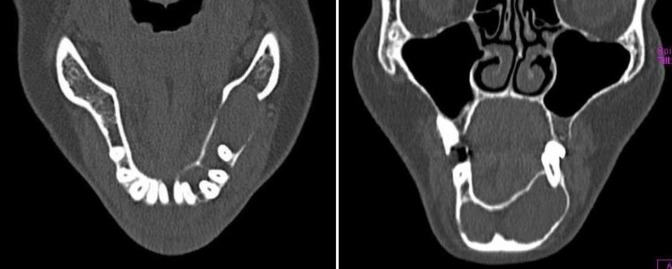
CT scan showed an expansile cystic lesion in the mandible, with expansion and thinning of both buccal and lingual cortices (Figures 5 and 6).
